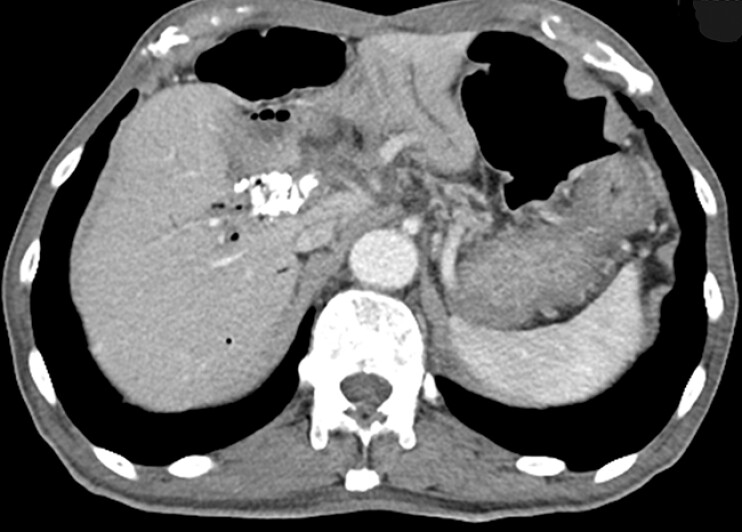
Contrast-enhanced computed tomography scan 3 months after the treatment showing the Histoacryl embolization of the varicose vein.
